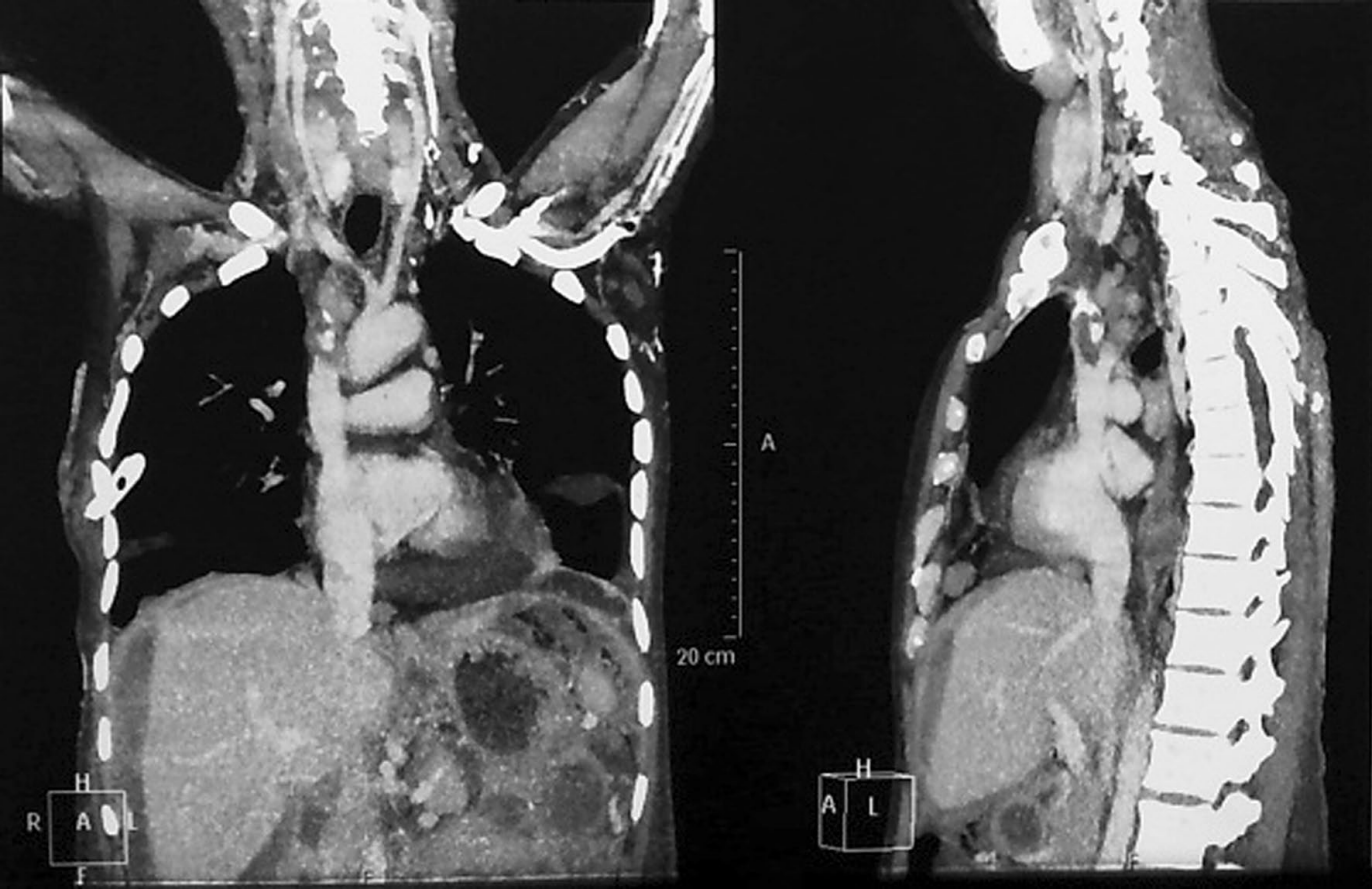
CT venography chest and abdomen showing hypodense superior vena cava and infra renal inferior vena cava suggestive of thrombosis with mid ascites with omental thickening
